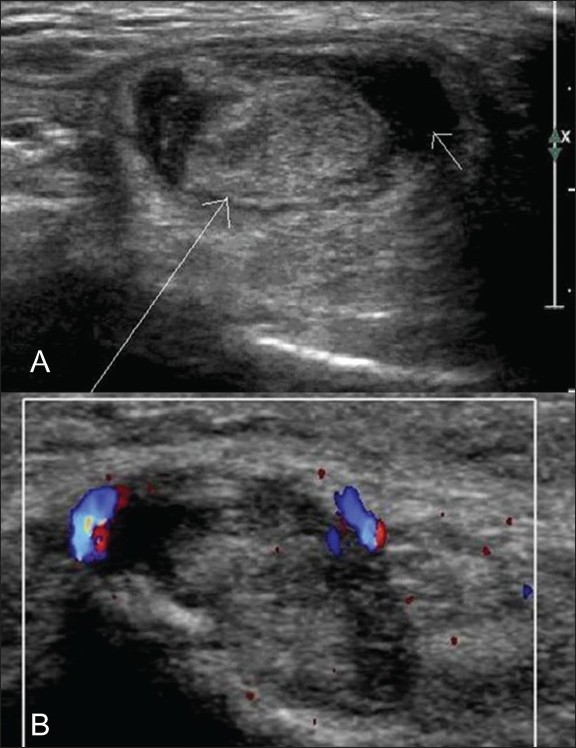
Tenosynovitis. Transverse gray-scale (A) and color Doppler (B) USG shows an edematous tibialis posterior (long arrow) with a small amount of fluid (small arrow in A) in the tendon sheath and increased vascularity on color Doppler
